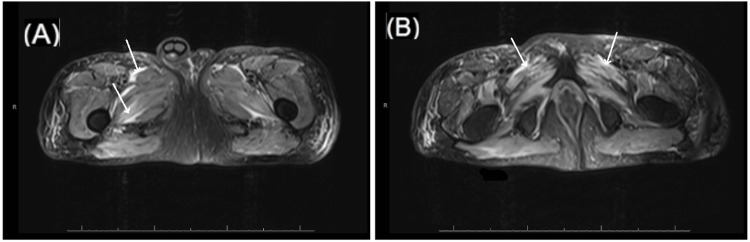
MRI Demonstrating Features of Myositis T2-weighted MRI images with contrast obtained from the patient: (A) arrows highlight hyperintensities of the adductor musculature without abnormal enhancement; (B) arrows highlight hyperintensities of the gluteus maximus musculature without abnormal enhancement. These areas of hyperintensity are consistent with patchy edema, which can be seen in myositis.
